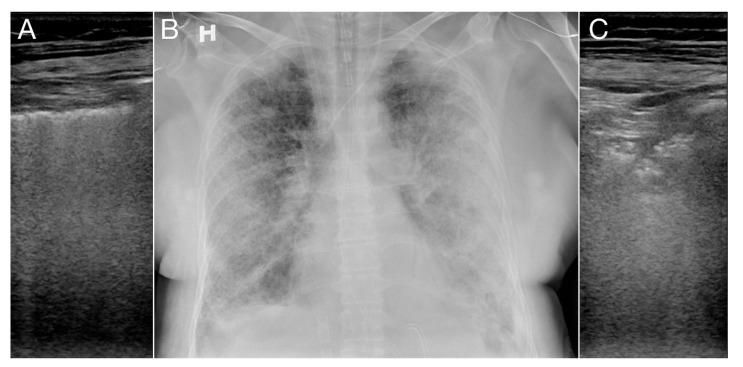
A patient with corresponding LUS and chest X-ray is shown. The ultrasound images are taken from the lateral view of each lung. The interstiel edema in the right lung visible with LUS as increased B-lines (A) is seen as numerous kerley B-lines in the chest X-ray (B). The consolidations with air-bronchograms in the left lung visible with LUS (C) are seen as subpleural consolidations in the chest X-ray (B).
